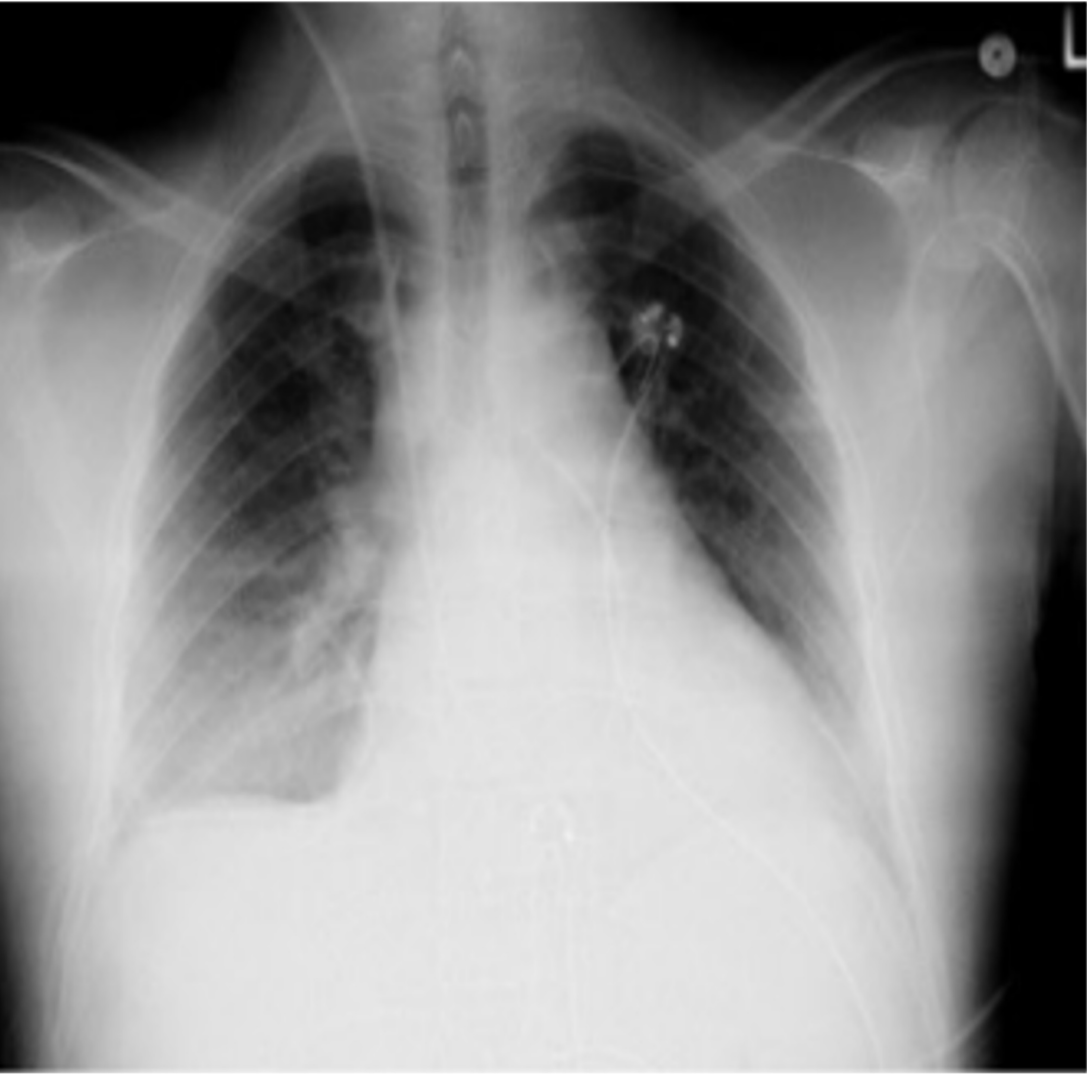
Chest X-ray showing pulmonary vascular congestion and right mid and lower lung opacity/effusion.
